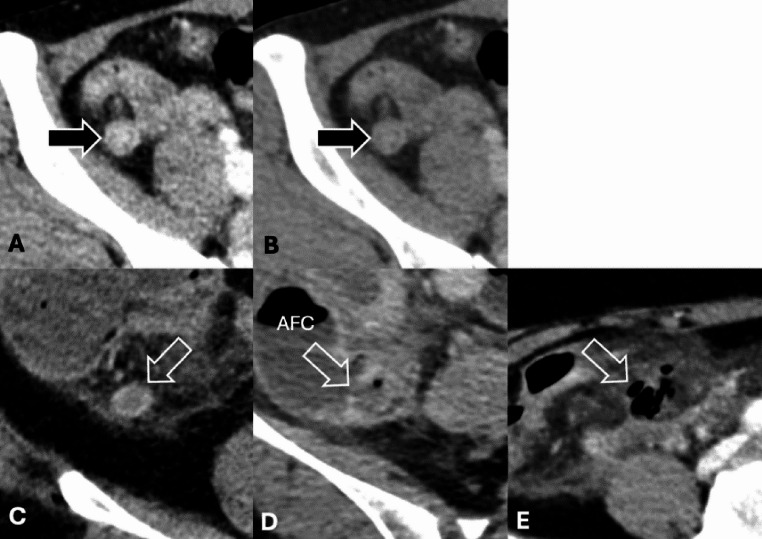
Wall enhancement patterns of acute appendicitis. Axial contrast-enhanced CT images (A: 50-keV virtual monoenergetic, B: 120-kVp-equivalent) show smooth, uninterrupted enhancement of the wall (black arrows). Note an apparent increase in iodine attenuation on the low-keV image, relative to conventional image. Figure 2C, D and E are 50-keV images of three patients depicting interrupted appendiceal wall enhancement (i.e., defect; open arrows) due to nonenhancing wall, fluid in the wall, and air in the wall, respectively. *AFC* air-fluid collection
